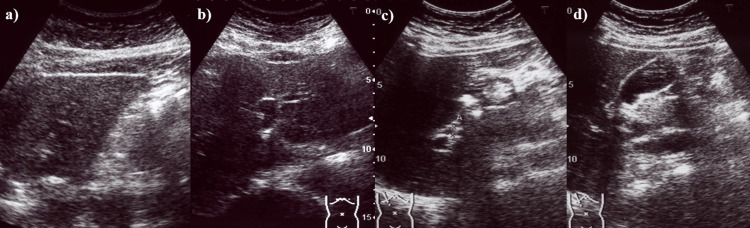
Abdominal ultrasonography (US) of the liver. (a) The liver had a dull edge. (b, c) No mass was detected in the liver, and no dilatations were seen in either of the intrahepatic bile ducts or in the common bile duct. (d) The gallbladder showed no abnormalities on imaging.
